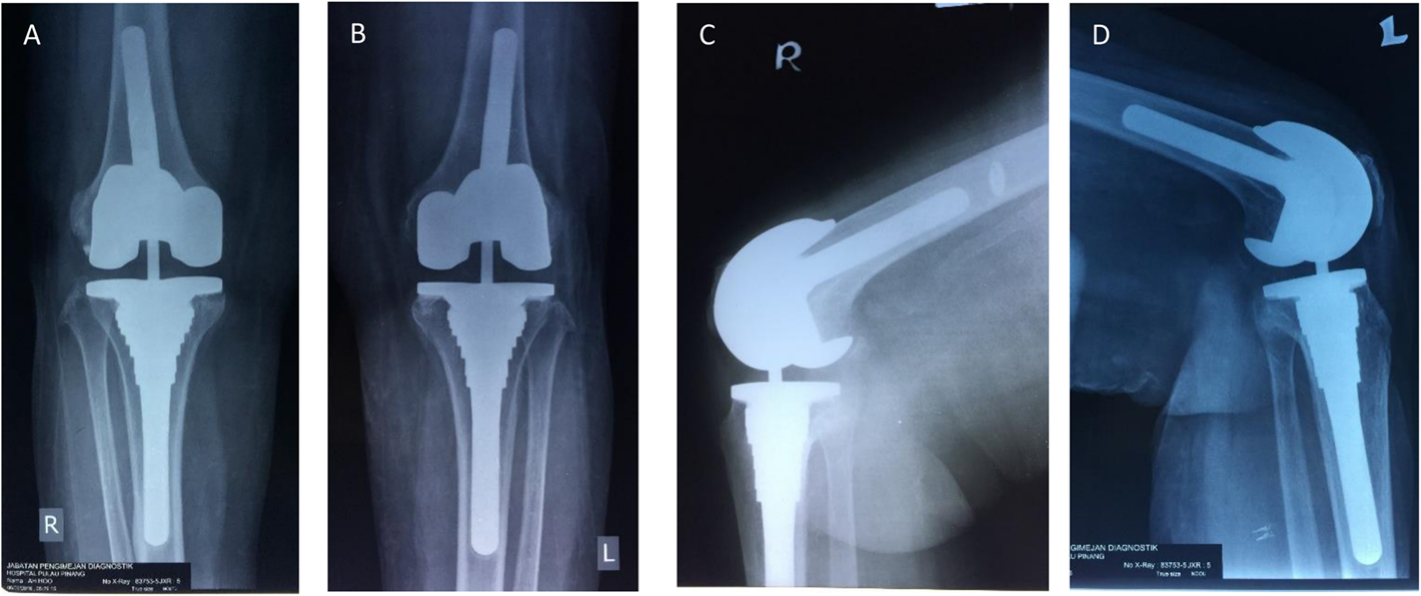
Post-op radiographs of one of our patients who had Type 2B defects bilaterally. a Anteroposterior (AP) Standing of Right knee. b Anteroposterior (AP) standing of left knee. c Lateral view of right knee. d. Lateral view of left knee
